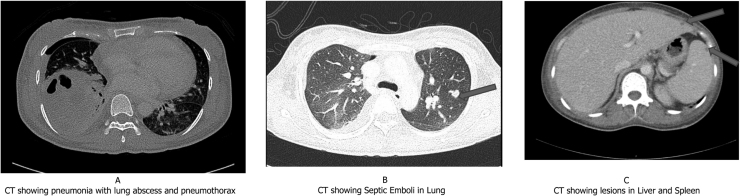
(A) CT showing pneumonia with lung abscess and pneumothorax (B) CT showing Septic Emboli in Lung (C) CT showing lesions in Liver and Spleen.
